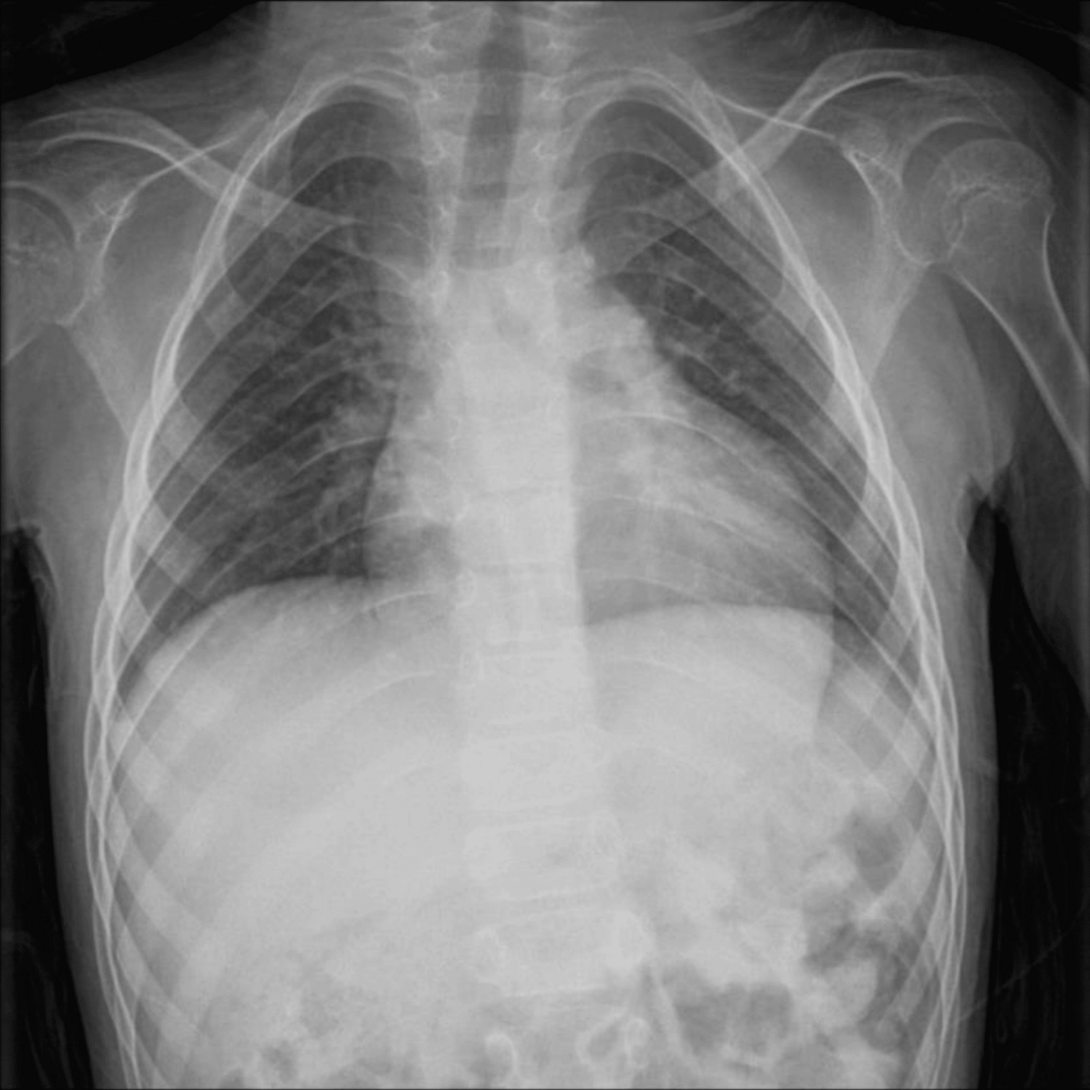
An antero-posterior chest radiograph of a nine-year-old patient with mucolipidosis type IV Chest radiograph demonstrating an increased cardiothoracic ratio (~58%), raising suspicion for cardiomegaly.
